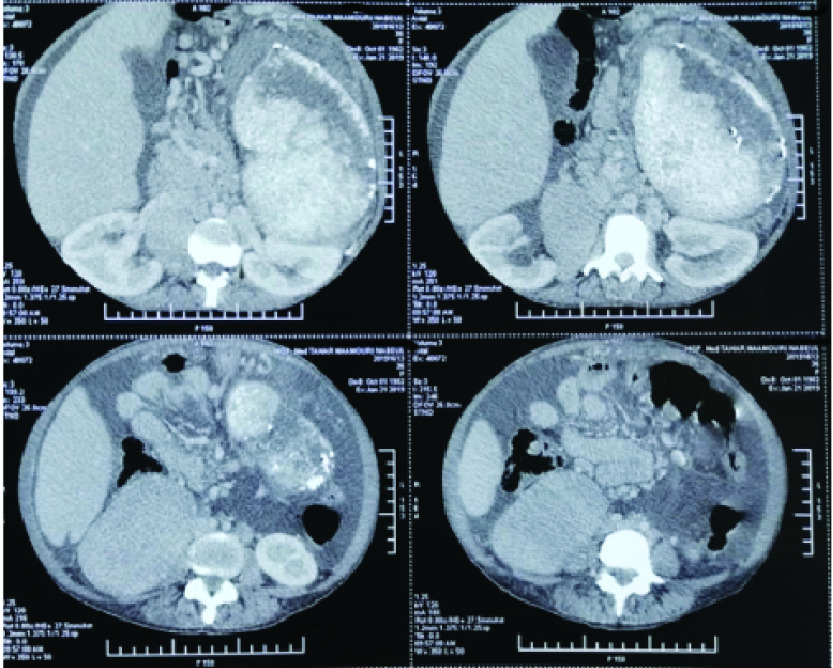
Computed tomography scan showing ascites, portosystemic collateral circulation, homogenous hepatomegaly, heterogeneous splenomegaly containing capsular and intrasplenic calcifications and segmental infarction areas. Computed tomography scan also shows a homogeneously enhanced right paravertebral mass measuring 14 × 9 × 7 cm.
